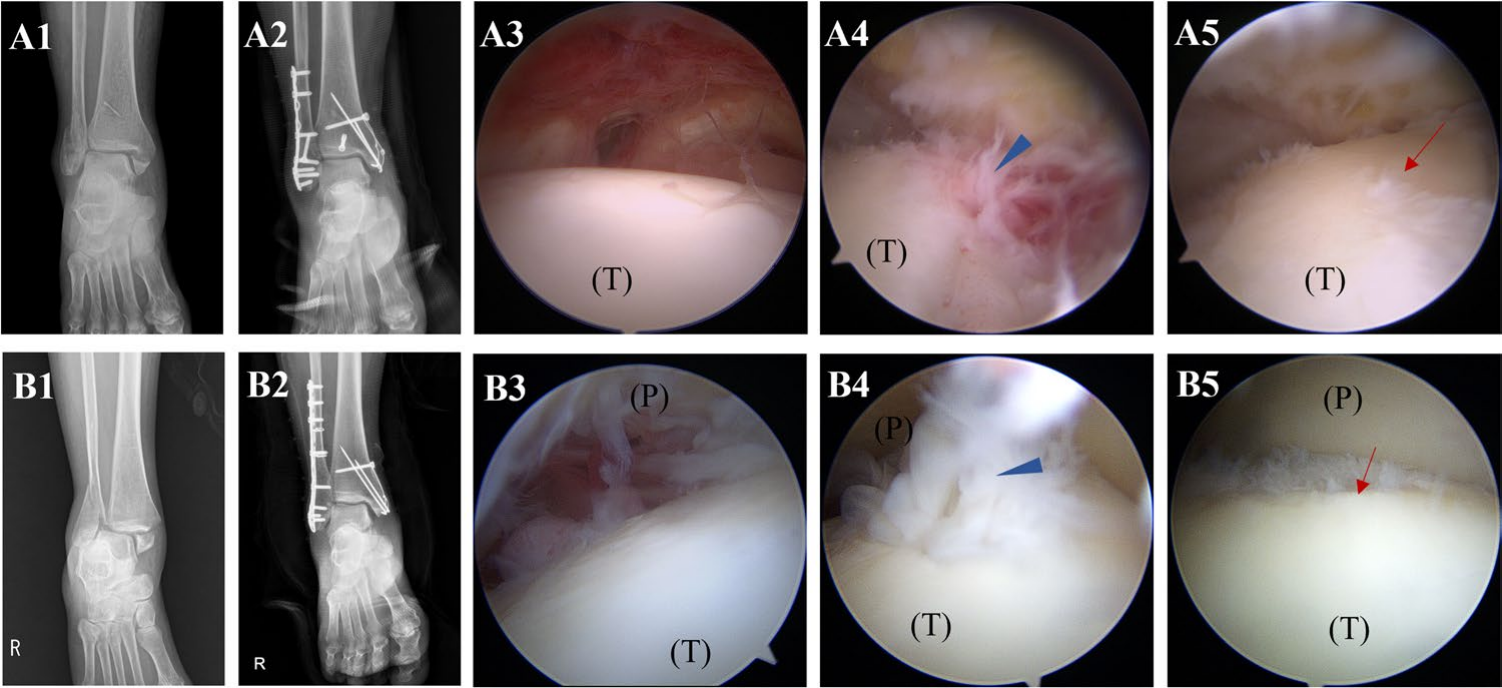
The second-look arthroscopic pictures show the growth of intra-articular scar tissue following trimalleolar fracture (A1–A5) and bimalleolar fracture (B1–B5). (A1, B1) Preoperative X-ray. (A2, B2) Postoperative X-ray shows good reduction and internal fixation. (A3, B3) The initial intact cartilage at fractured time. (A4, B4) The second-look arthroscopic finding depicts scar tissue (arrowhead) formed extensively and adhered to the talar dome. (A5, B5) The underlying degenerative cartilage damage (arrow) is exposed after removing scar tissue. (P): Tibial plafond, (T): Talus.
